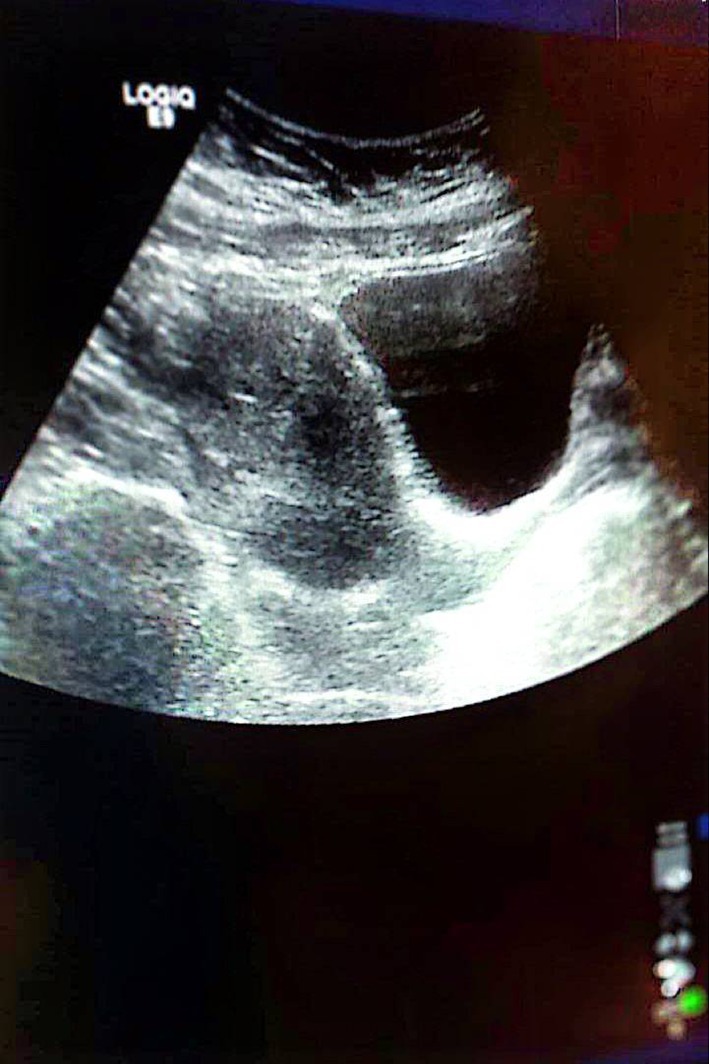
Two‐year follow‐up pelvic ultrasound performed with a half‐full bladder. The uterus is anteverted and positioned anteriorly. Uterine size and morphology are within normal limits. Both ovaries and adnexal structures appear normal, with no evidence of masses, cysts, or abnormal fluid collections.
